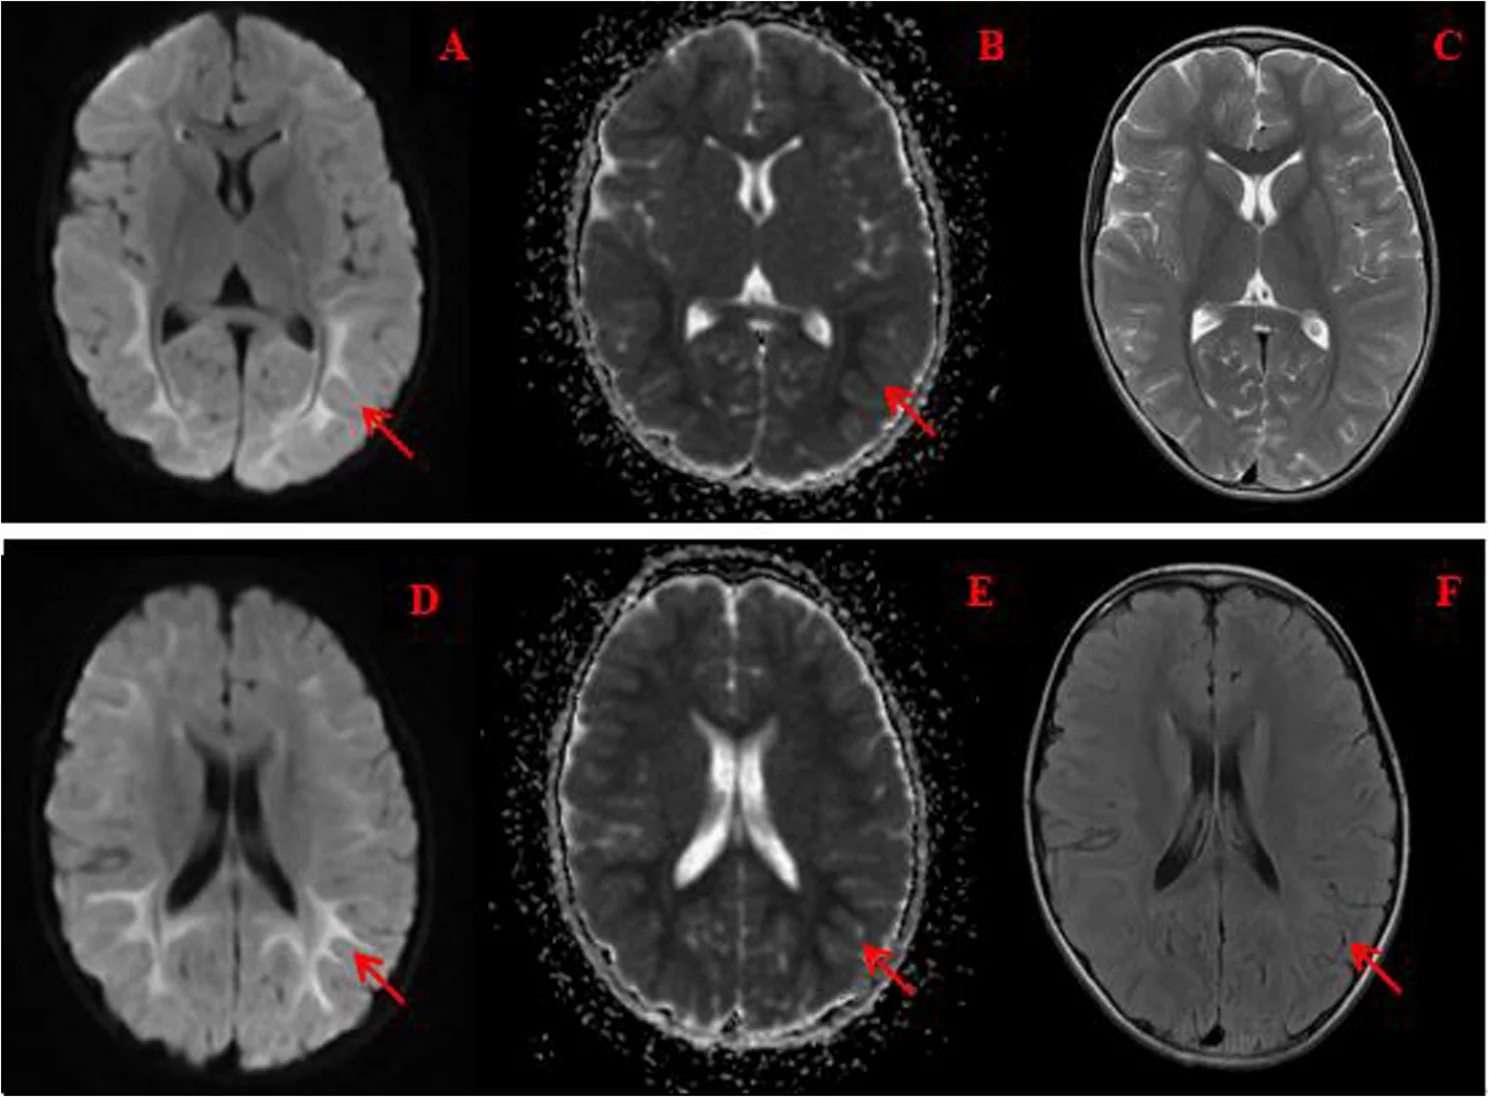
Brain MRI results of the child with hypersarcosinemia (June 13, 2025, four and a half months after discharge). A and D: DWI hyperintense signal in the subcortical white matter of the bilateral cerebral hemispheres was observed, and the degree of intensity increase was slightly reduced (arrows). B and E: Hypointense signals on ADC maps in the subcortical white matter of the bilateral cerebral hemispheres (arrows). C: The T2-weighted image showed no abnormalities. F: Slight FLAIR hyperintensity in the white matter of the posterior horn of the bilateral ventricles (arrow). MRI, magnetic resonance imaging. DWI, diffusion-weighted imaging. ADC, apparent diffusion coefficient. FLAIR, fluid-attenuated inversion recovery
